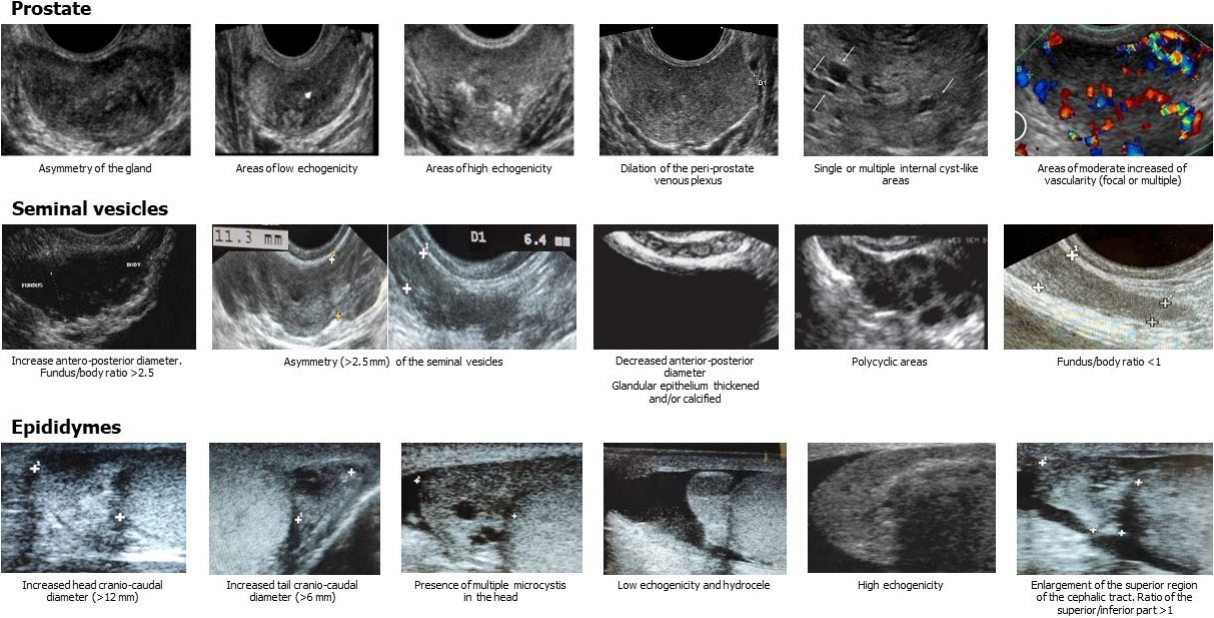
MAGI Diagnostic Criteria: Ultrasound Panel
